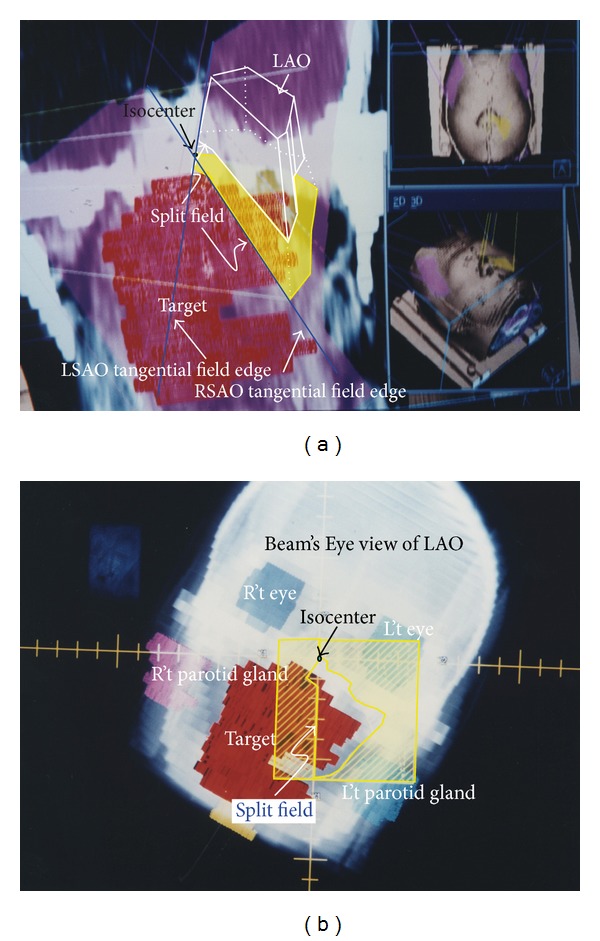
(L't) Left anterior oblique (LAO, yellow color area) field was used to compensate the target dose deficient at left side where it is shown outside the “A” shape at upper right on this figure. The yellow color wash region represents the field aperture of LAO on coronal plane and on patient surface. The central axis plane abutted the interface of “A” shape and LAO, which was created by rotating the collimator to 153°, gantry angle to 180°, and couch rotation to 180°. Forty-five degree wedge with “big head” toward R't side was added for dose uniform modification. (R't) This picture shows the Beam's Eye View (BEV) in Digital Reconstruction Radiograph (DRR) of LAO field. This field was created essentially for compensating the target dose deficiency right side to “A” shape. The interface close to “A” (denoted as “split” abutted onto A shape edge on this figure) was opened by jaw to form nondivergence field.
